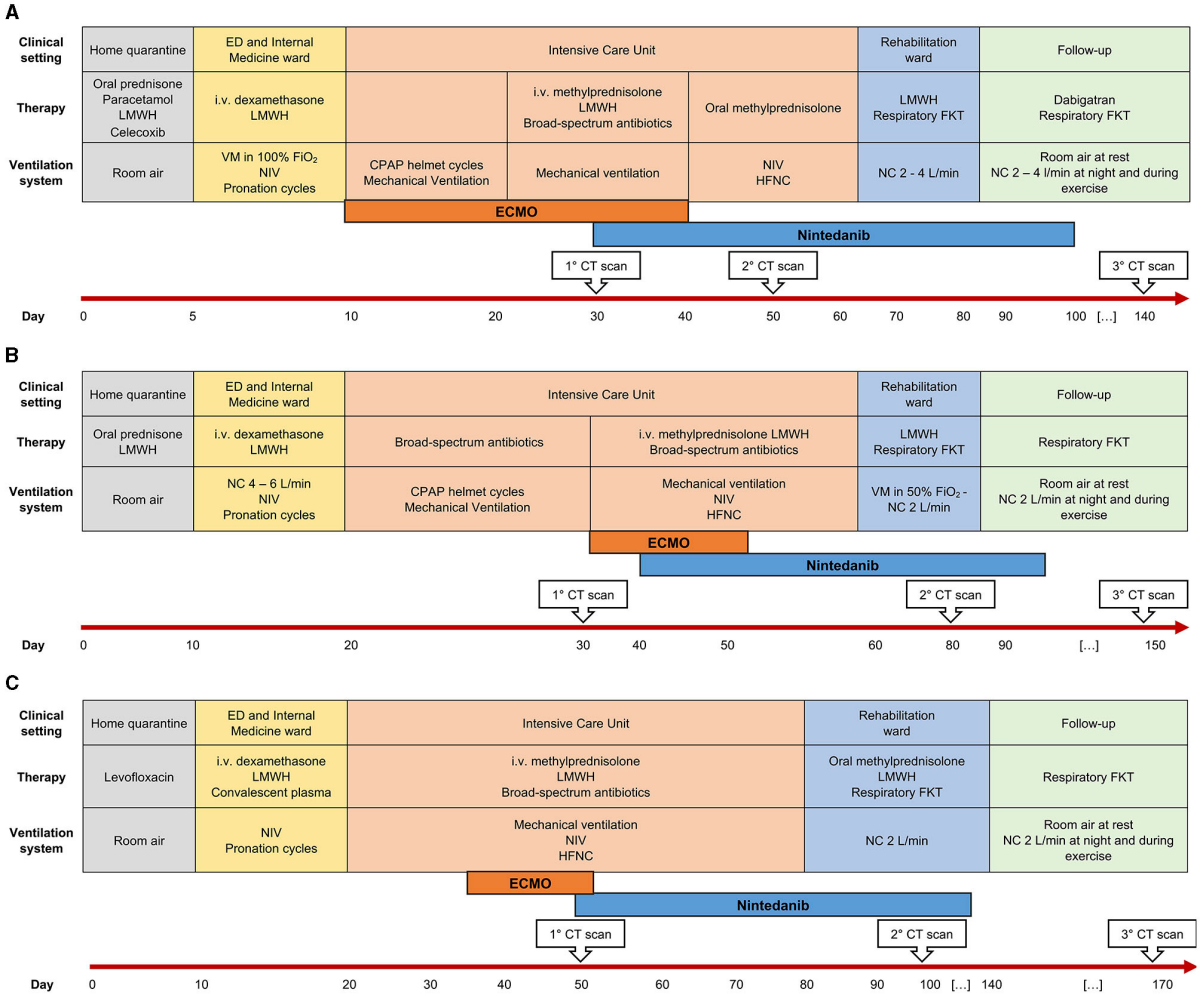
Disease course and patient management over time in three patients (A–C). ED, Emergency Department; LMWH, low-molecular weight heparin; i.v., intravenous; VM, Venturi mask; FiO_2_, fractional inspired oxygen; NIV, non-invasive ventilation; CPAP, continuous positive airway pressure; HFNC, high-flow nasal cannulae; FKT, physiokinesitherapy; NC, nasal cannulae; CT, computed tomography; ECMO, extra-corporeal membrane-oxygenation.
